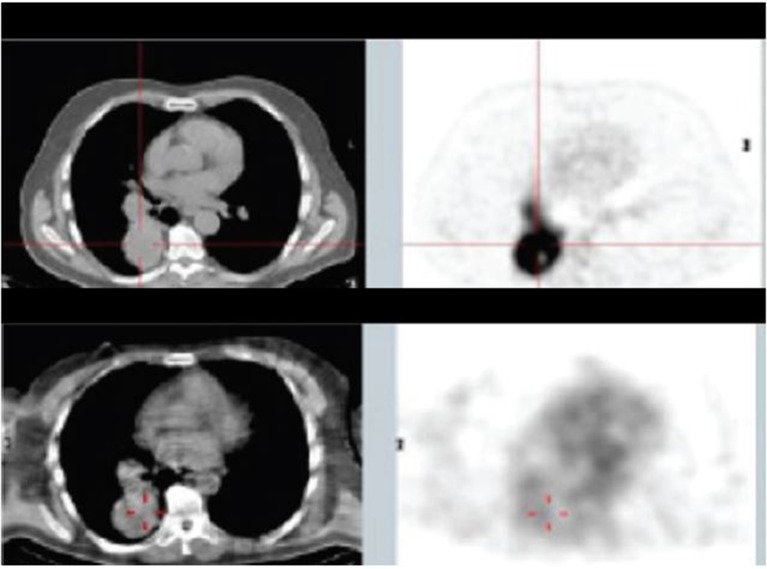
Patient from Phase Ia study with mediastinal and lung metastases: top panel—^18^FDG PET/CT 10 days before the study, lower panel—SPECT/CT of ^188^Re-6D2 mAb at 2 h after injection [from Klein et al. (156), available under the terms of the Creative Commons Attribution (CC BY)].
